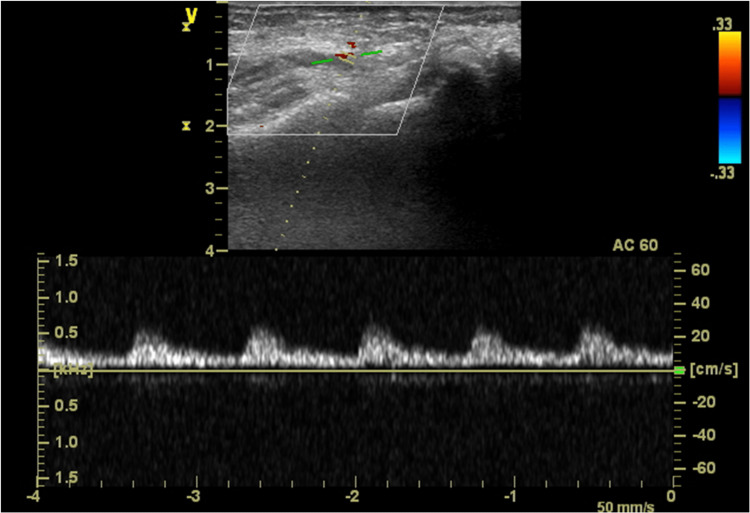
Right radial artery flow with ultrasonography post spasm and removal of the vascular sheath
